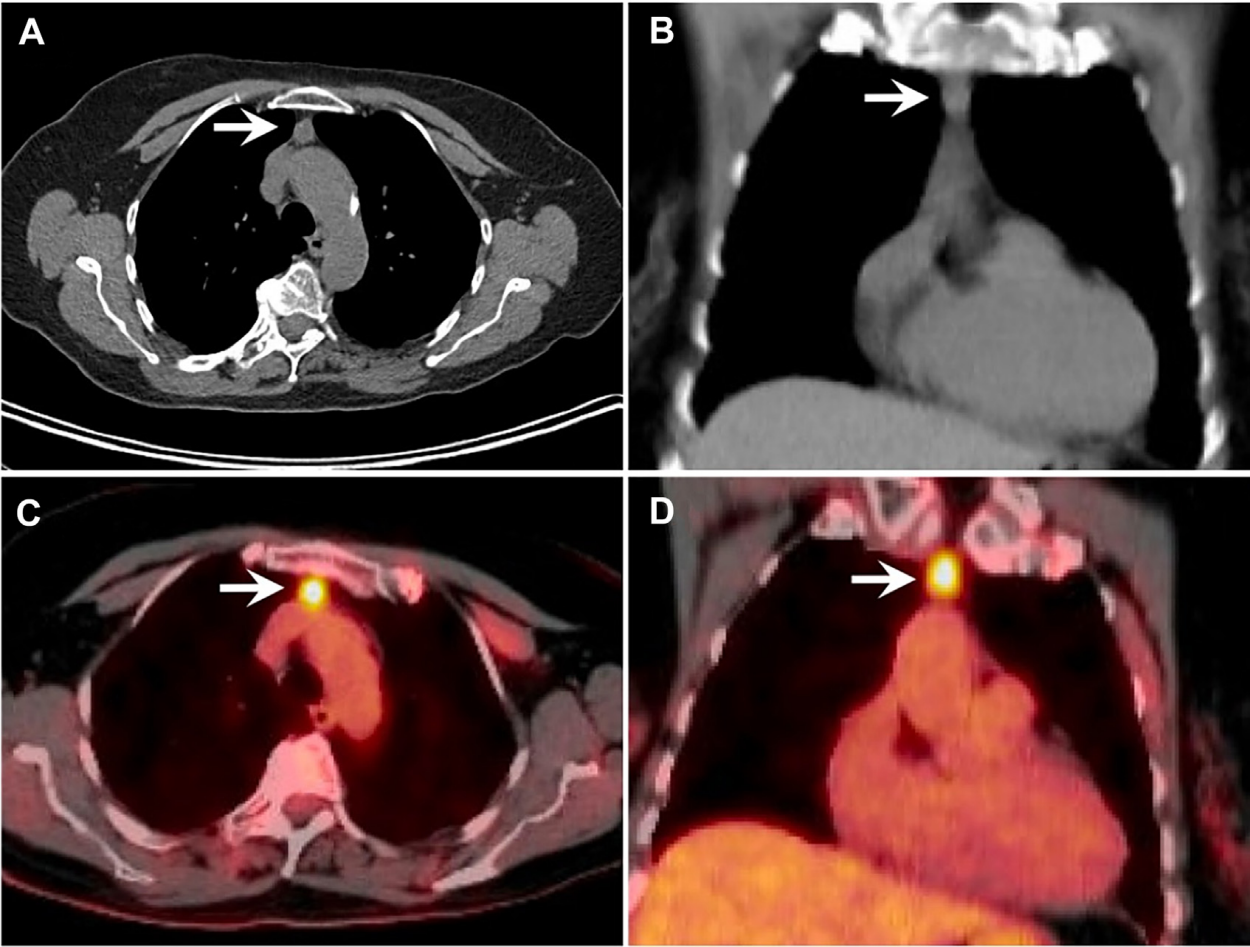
(A, B) Preoperative non–contrast-enhanced chest computed tomography scan demonstrates soft tissue calcification in the anterior mediastinum (arrow) with (C, D) positron emission tomography/computed tomography imaging showing metabolic hyperactivity in the thymus (arrow).
